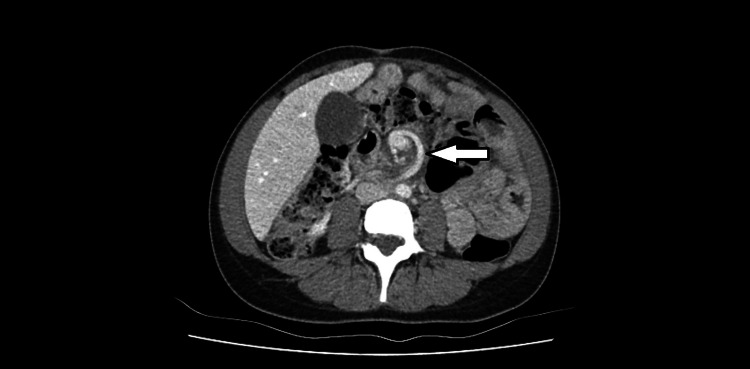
An axial CT image showing whirling of the small bowel and mesenteric vessels around superior mesenteric vein leading to its congestion
